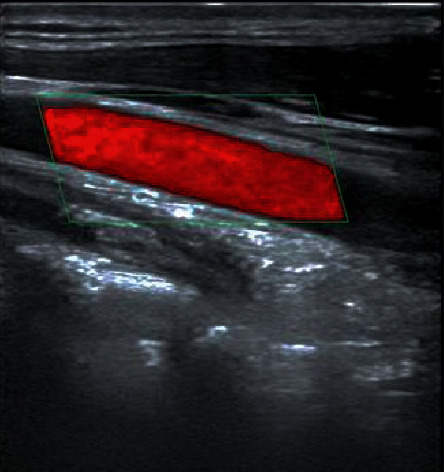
Colour Doppler image of the anterior tibial artery.
